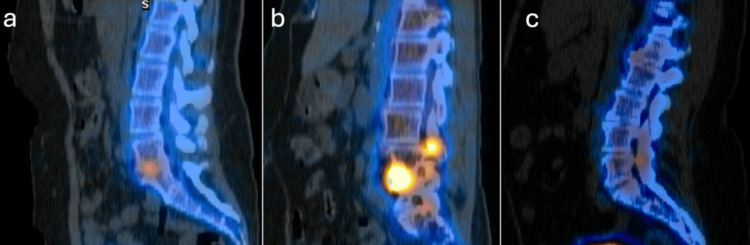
Examples of different SPECT-CT signatures a) Disc space only: 48-year-old female. Single Photon Emission Computed Tomography-Computerized Tomography (SPECT-CT) dominant uptake at the L5/S1 disc space and had a baseline visual analogue scale (VAS) pain score of 7. After an L5/S1 anterior lumbar interbody fusion (ALIF), she reported a VAS pain score of 2 at one year postoperatively. b) Disc space and facet joint: 69-year-old. SPECT-CT dominant uptake at L4/L5 disc space and L3/4 facet joint with a baseline VAS pain score of 6. She underwent an L3-L5 lateral interbody fusion and reported a VAS pain score of 4 at one year postoperatively. c) Facet joint only: 58-year-old female. SPECT-CT dominant uptake at the L4/L5 facet joint and had a baseline VAS pain score of 7. She underwent an L4/L5 prone trans-psoas approach interbody fusion along with L4/L5 posterior instrumentation and reported a VAS pain score of 6 at one year postoperatively.
